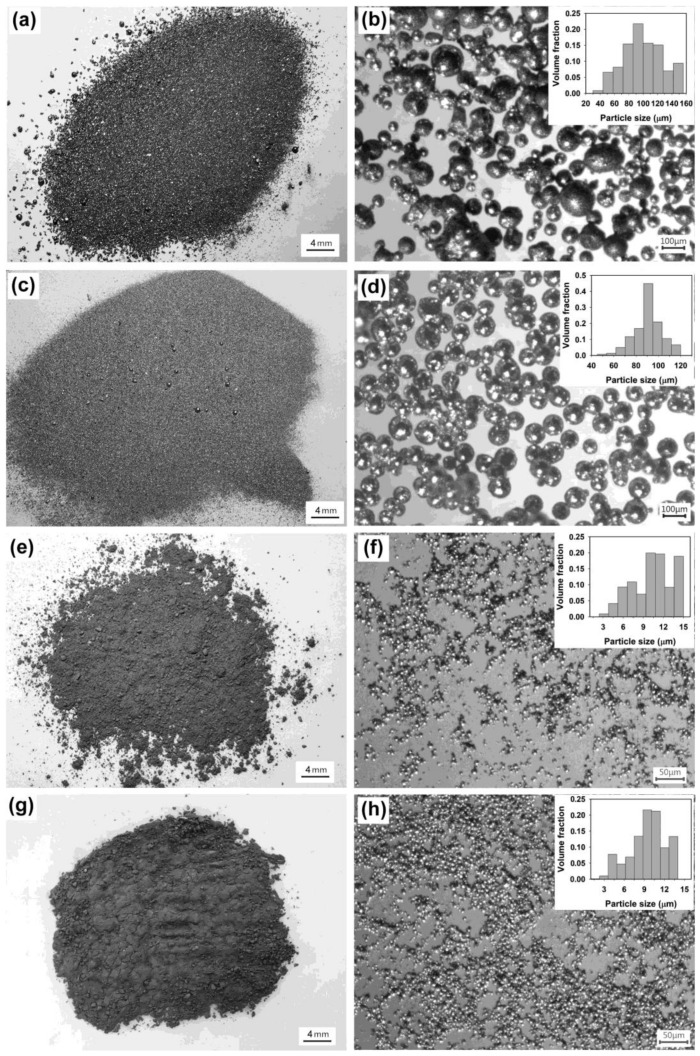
Optical images of the products obtained under different irradiation times: (a,b) 1 min, (c,d) 2 min, (e,f) 10 min, and (g,h) 15 min. Other experimental conditions: ultrasonic power = 1080 W and volume ratio of salts to metal = 25:1. Inset is the corresponding volume-based particle size distribution.
